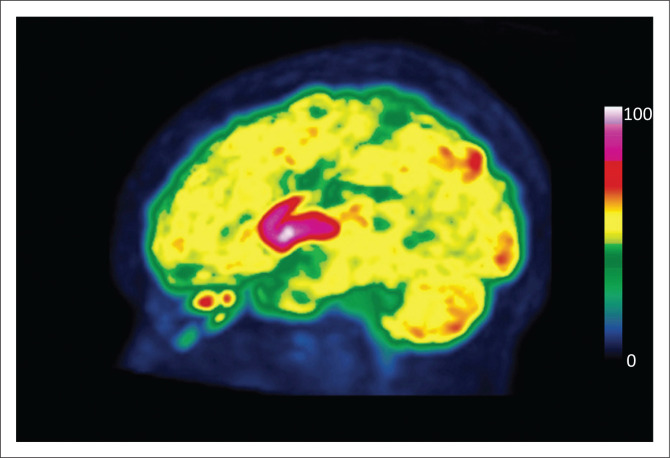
Fluorine-18 FDG PET (maximum intensity projection) in a patient known with systemic lupus erythematosus who presented with new-onset of choreoathetoid movements. Intense, symmetrical uptake in the striatal nuclei is consistent with a known neurological manifestation of systemic lupus erythematosus. MRI in this case reported non-specific features of microangiopathy.
